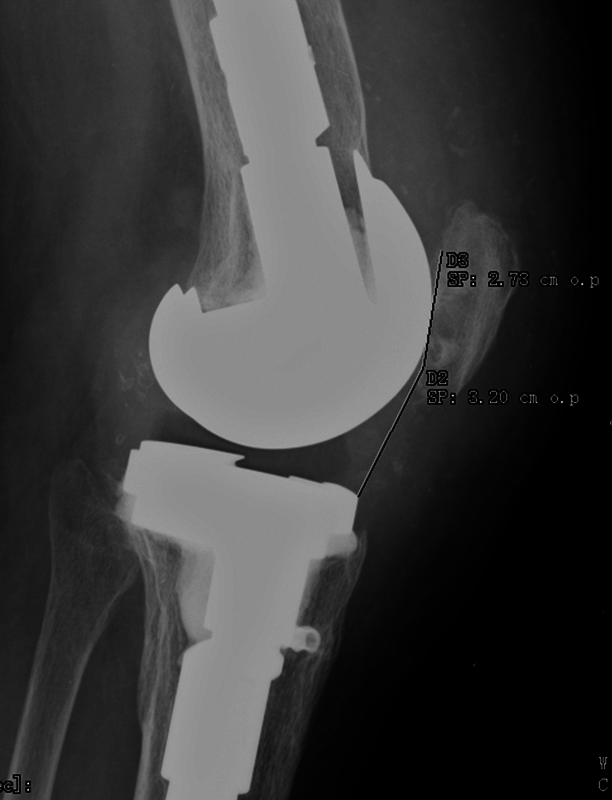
Lateral radiograph of the knee showing a Caton-Deschamps index of 1.17 postoperatively.
